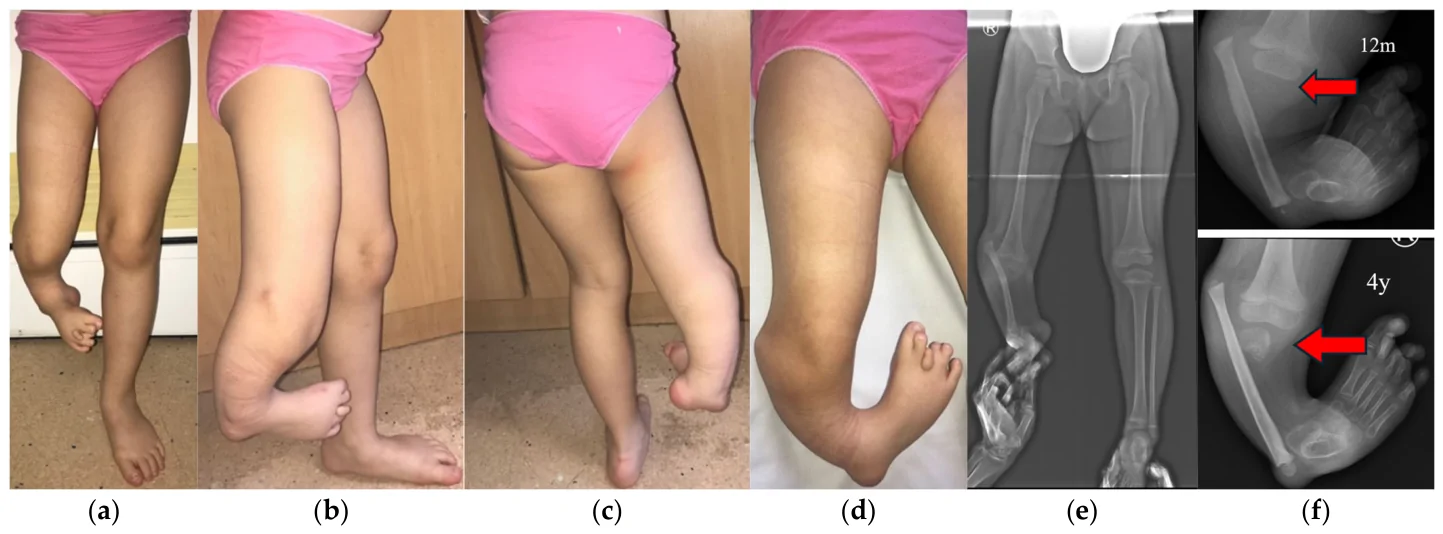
Clinical and radiographic views of right-sided type IVB TH: (a–d). Severe shortening, knee instability (e), equinovarus foot deformity, polydactyly (f). Radiographic view at 12 months and at 4 years of age presenting delayed epiphyseal ossification, and fibular over-growth. Red arrow is pointing the nucleus of ossification at the age of 12 months and 4 years. R—right.
